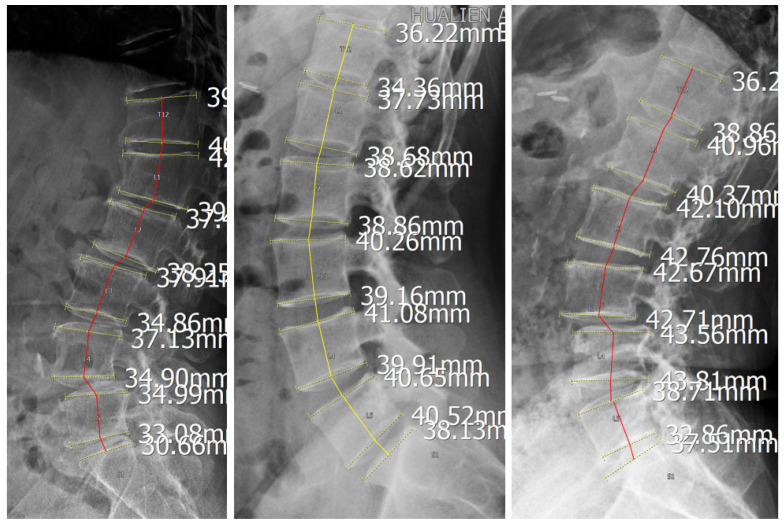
Construction of the lumbar lordotic curve on sagittal radiographs.  Figure 2 shows the construction of the lumbar lordotic curve on a sagittal lumbar radiograph by sequentially connecting the centroids of the vertebral bodies from T12 to S1.
